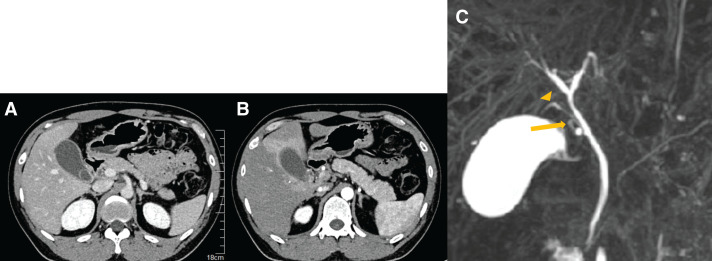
Contrast-enhanced CT scan findings at initial presentation. Contrast-enhanced abdominal CT revealed a 20-mm gallstone impacted in the gallbladder neck (A), with gallbladder distention and mild tension. The gallbladder wall was diffusely edematous, and early inflammatory changes were observed at the gallbladder bed (B). MRCP revealed that the cystic duct (arrow) drained into an AHD, specifically the bile duct from segment 6 (arrowhead) (C). AHD, accessory hepatic duct; MRCP, magnetic resonance cholangiopancreatography
